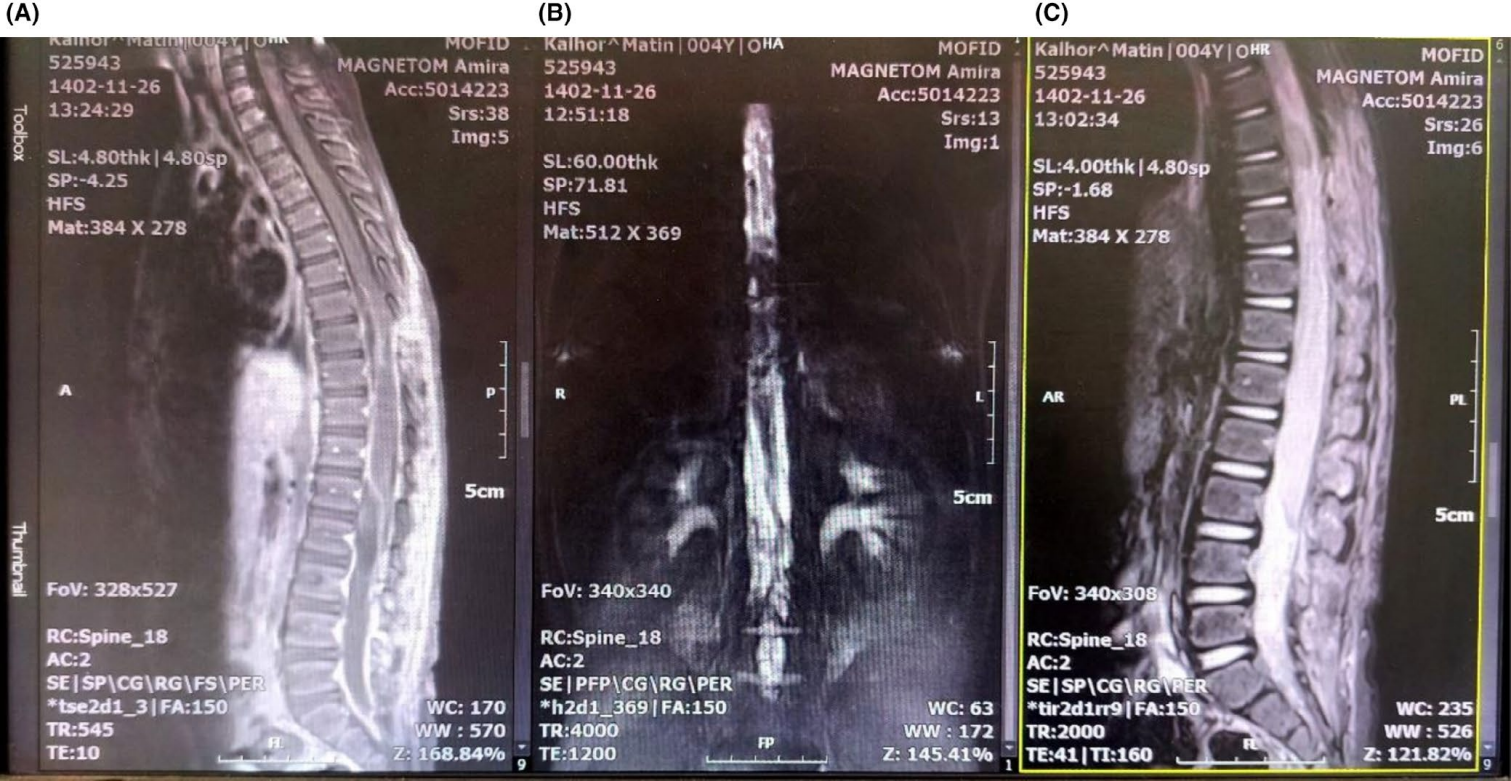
Early postoperative thoracolumbar imaging. (A) T1‐weighted MRI with gadolinium injection (left), (B) Myelogram (middle), (C) T2‐weighted MRI with gadolinium injection (right). They depict no residual hydatid cysts.
